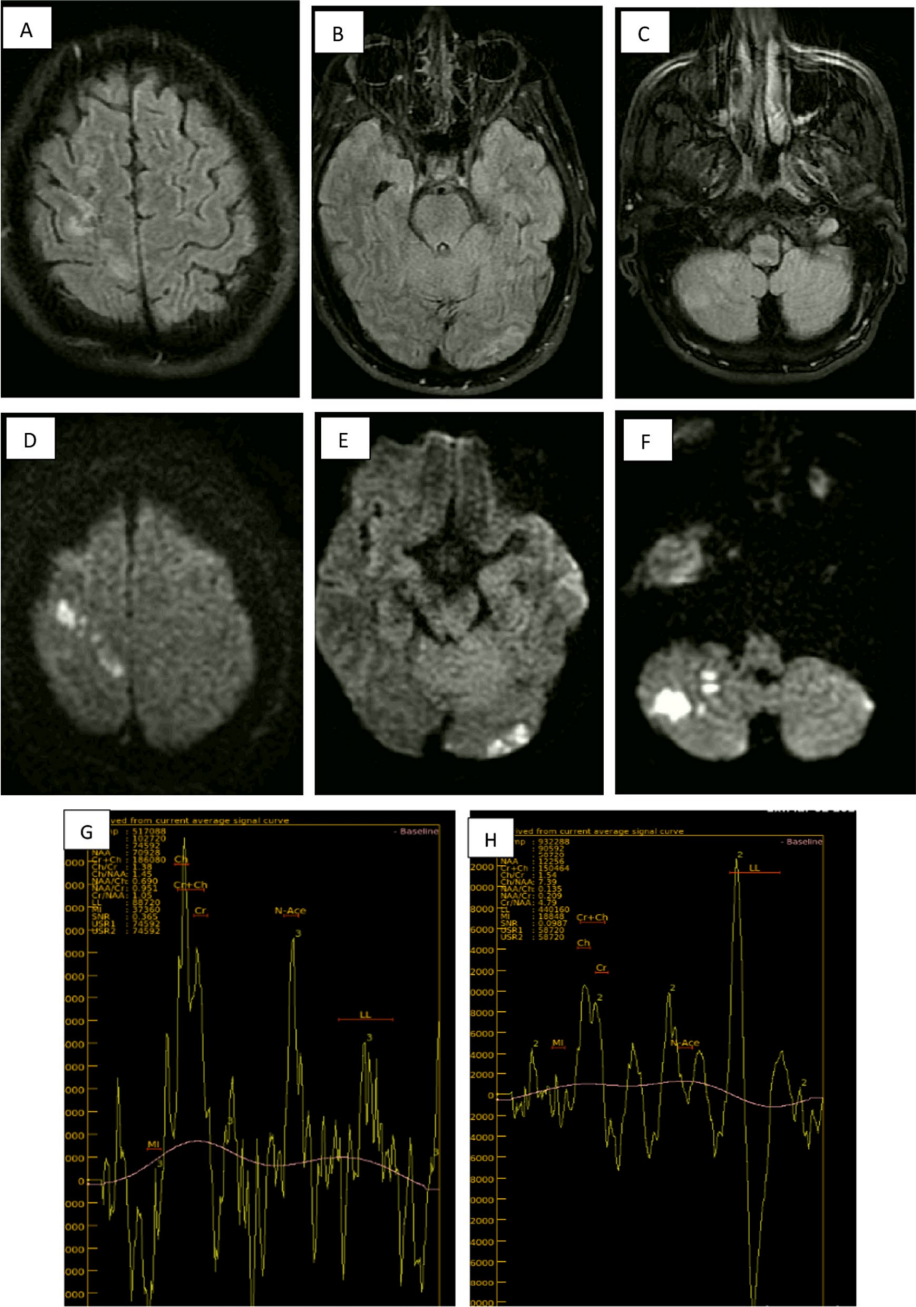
A 39-year-old male with dyspnea and fever, 6 days later, he presented with disturbed gait, weakness of both lower limbs and dysarthria. A Axial FLAIR sequence at high parietal regions, B axial FLAIR at parieto-occipital regions showing areas of altered high signal intensity, C axial FLAIR at both cerebellar hemispheres levels showing right cerebellar areas of altered high signal intensity. D Axial DWI at high parietal level, E axial DWI at parieto-occipital level, F axial DWI at both cerebellar levels showing restricted diffusion of the previously mentioned areas of altered high FLAIR signal intensity. G Long TE(144) of MRS, showing mild decreased NAA, creatine, myoinositol, mild elevated choline peak, H short TE(35) of MRS showing shooting of lipids/lactate peaks (consistent with necrosis and anaerobic metabolism). Diagnosis was multiple non-hemorrhagic infarctions. Diagnosis: multiple recent infarctions along both PCA and right posterior-inferior cerebellar artery territories)
